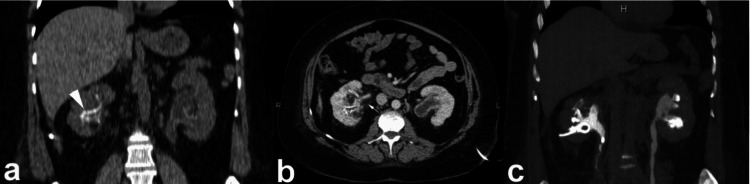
Non-enhanced CT (NECT) scan (a), a contrast-enhanced CT (CECT) scan in the nephrographic phase (b), and a urographic phase (c). The NECT scan (a) reveals wall calcifications within the pelvicalyceal systems and proximal ureters, as denoted by the arrowheads. Subsequent imaging with CECT during the nephrographic phase (b) provides enhanced visualization of the inflammatory changes within the pelvicalyceal systems and proximal ureters. Collectively, these imaging findings support the diagnosis of encrusted uretero-pyelitis. There are also multiple calculi showcased during the excretory phase CECT. This figure is a republished image, permission was obtained from the original publisher, https://epos.myesr.org/poster/esr/ecr2018/C-2802
